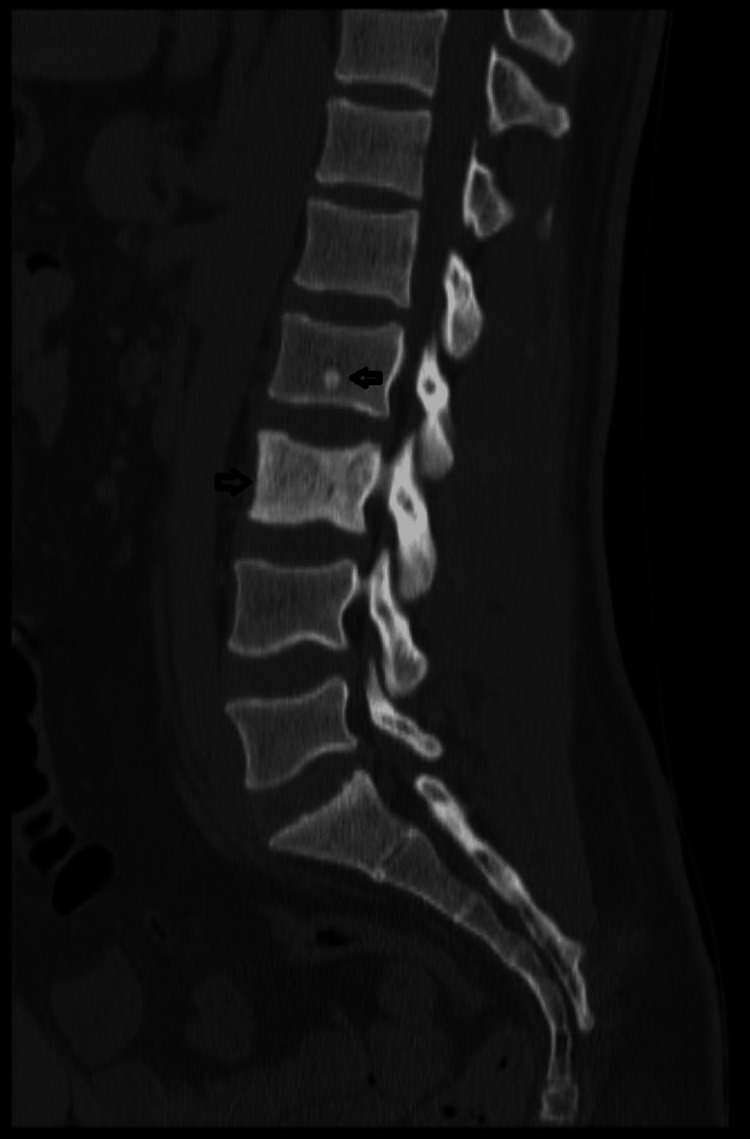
Sagittal section of the lumbosacral spine with metastases in L2 and L3 on plain CT scan
